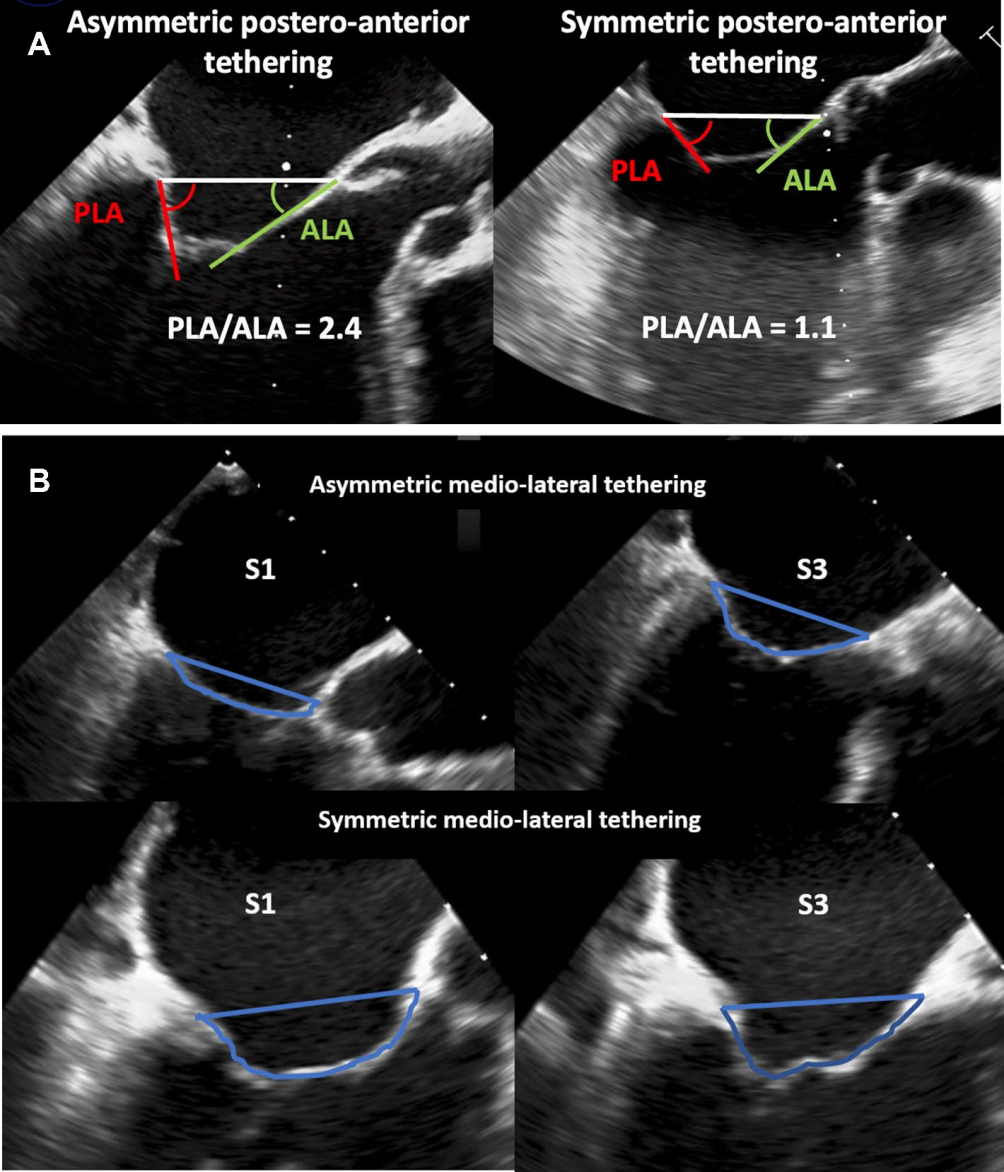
Symmetric and asymmetric postero-anterior and medio-lateral MV leaflet tethering. A Transoesophageal echocardiography of a patient with asymmetric postero-anterior tethering (left) and a patient with symmetric postero-anterior leaflet tethering (right). B Transoesophageal echocardiography of a patient with asymmetric medio-lateral leaflet tethering (top) and a patient with symmetric medio-lateral leaflet tethering (bottom). *MV* mitral valve; *S* segment
